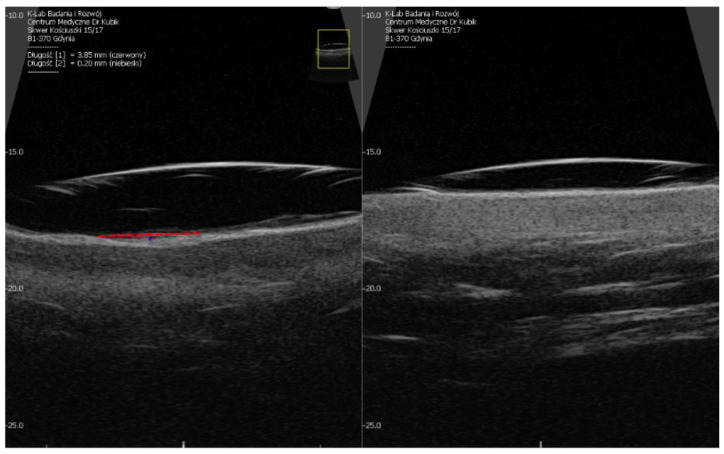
Representative high-frequency ultrasound (HFUS, 48 MHz) images of an atrophic acne scar before (left) and after (right) the combined treatment protocol. Prior to treatment, the scar depression is clearly delineated, with a transverse diameter of 3.85 mm (red marker) and a depth of 0.20 mm (blue marker). The ultrasound image was acquired with the depth scale displayed in millimetres, ranging approximately from 10 to 25 mm. At day 139, the scar contour is no longer discernible, consistent with structural normalisation of the dermal profile. A subtle difference in dermal echogenicity at the previously affected site reflects ongoing tissue remodelling.
